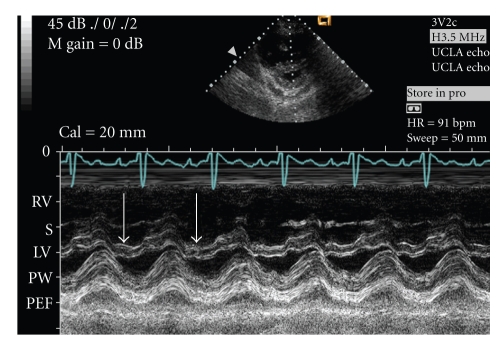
M-mode echo, parasternal short-axis, demonstrating leftward displacement of the septum during diastole (white arrows), consistent with right ventricular volume overload. Right ventricle (RV), septum (S), left ventricle (LV), posterior wall (PW), and pericardial effusion (PEF).
